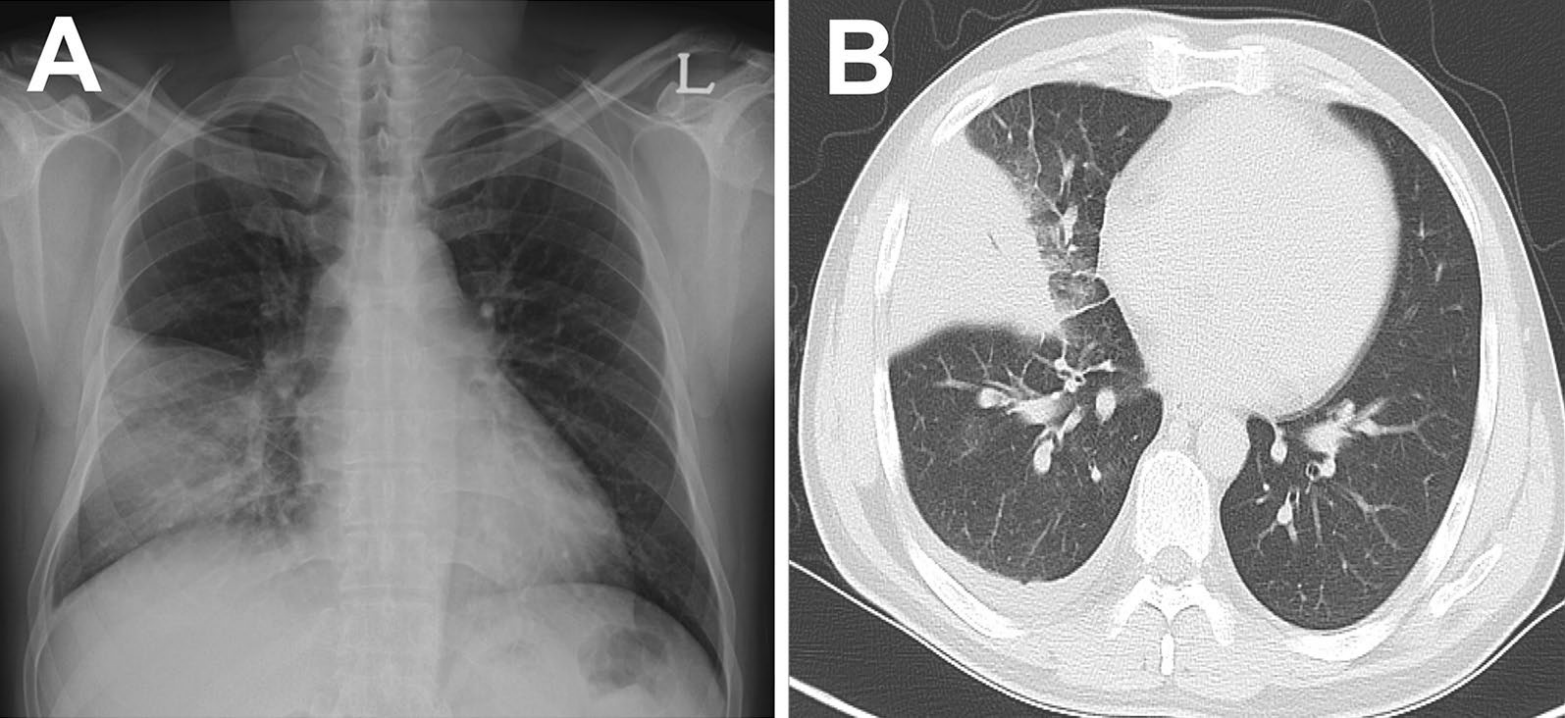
Chest images at presentation. Chest X-ray showed a consolidation in the right mid to lower lung fields (A). Chest computed tomographic scan showed dense consolidation with surrounding ground glass opacities in the lateral segment of the right middle lobe and a small amount of pleural effusion in the right hemithorax (B)
